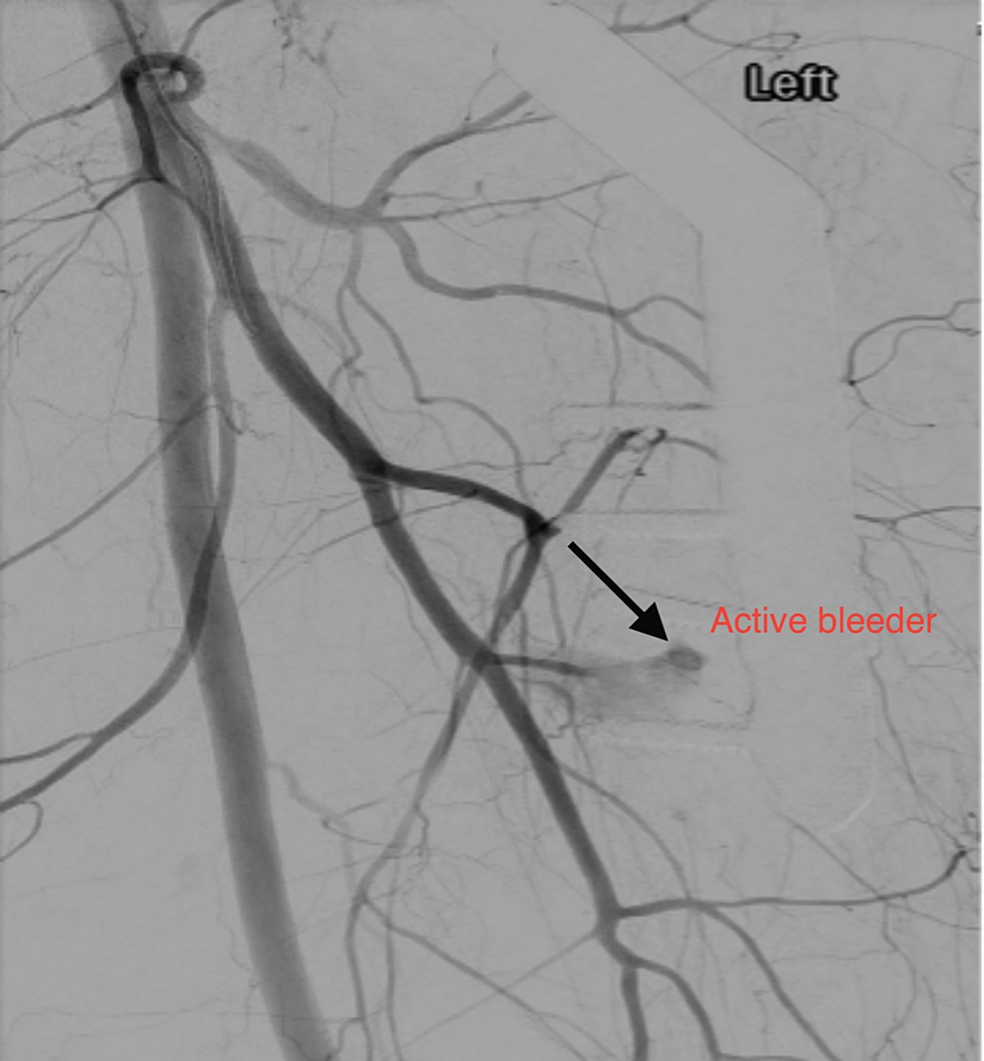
Active bleeding from one of the branches of the profunda femoris artery on CT angiogram of the left lower limb.
